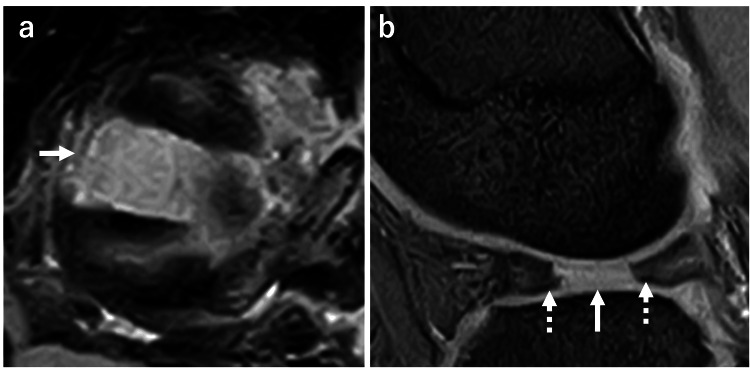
Preoperative MRI (a) Axial view showed a radial tear of the midbody of discoid lateral meniscus with an 11-mm-wide diastasis (white arrow) (b) Sagittal view showed a radial tear of the midbody and little degenerative change in the tear site (white dotted arrows)
